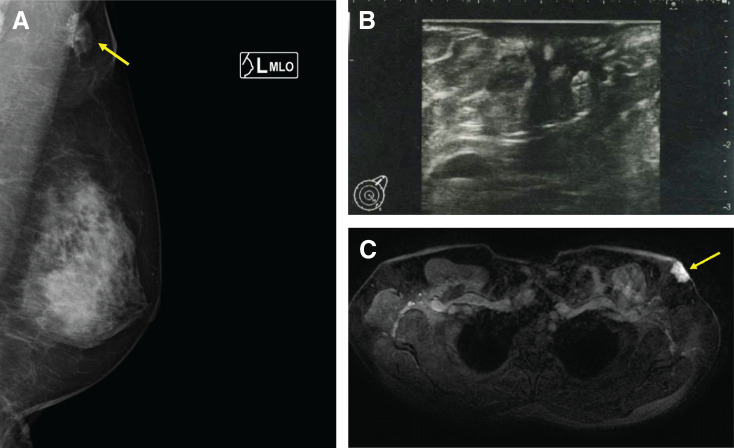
Imaging findings. (A) Mammography reveals a finely serrated, isodense mass (indicated by arrow) in the left axillary region. (B) US examination reveals an irregular hypoechoic mass measuring 14 × 12 × 11 mm with dermal invasion in the left axilla. (C) Breast contrast-enhanced MRI reveals a 20-mm enhancing nodule (indicated by arrow) in the left axilla.
